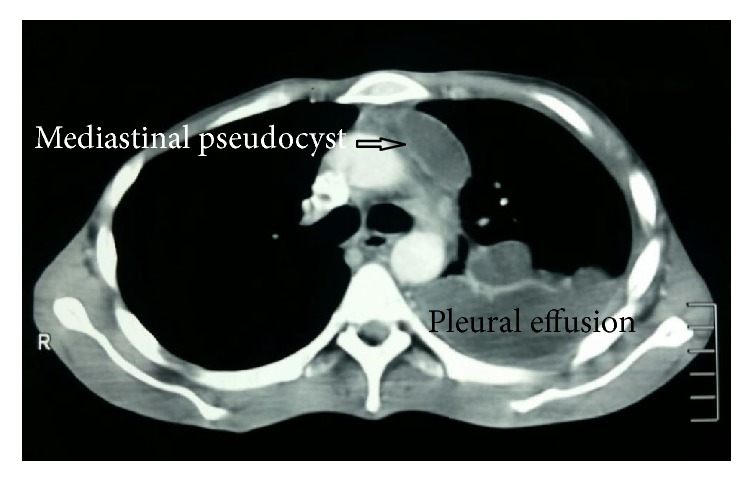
CT chest demonstrating mediastinal pseudocyst of size 3 cm, associated with left pleural effusion.
